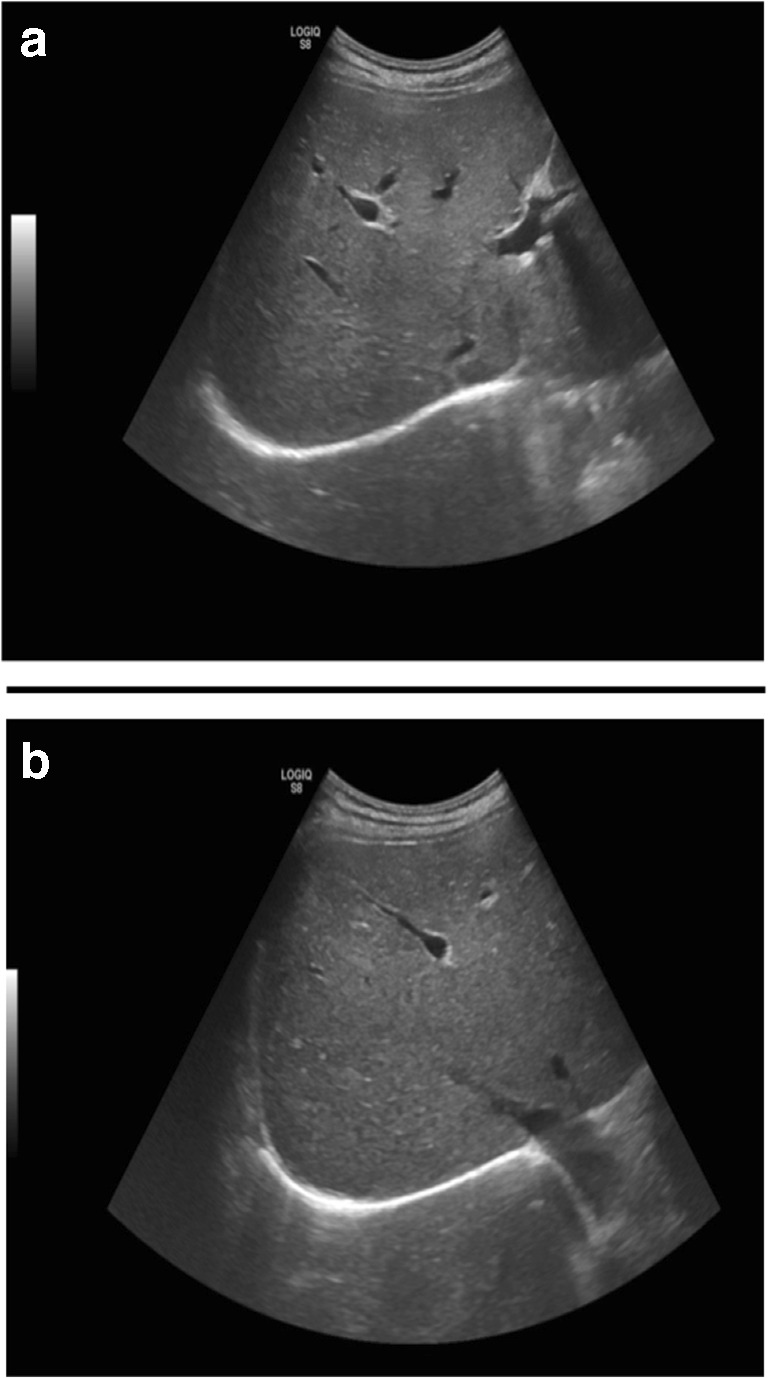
Hepatic ultrasound for patient 5 in a 2014 and b 2019 (unpublished data)
